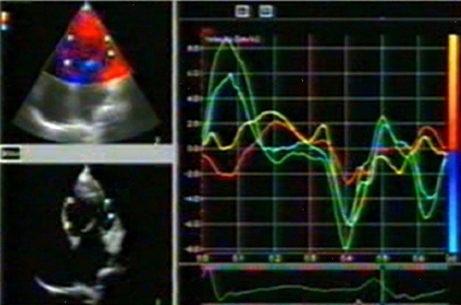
Tissue Doppler Image in a patient with Heart Failure and LBBB. Tissue Velocity curves corresponding to the sample volumes in the Lateral wall show a significant delay compared to the septal wall.
